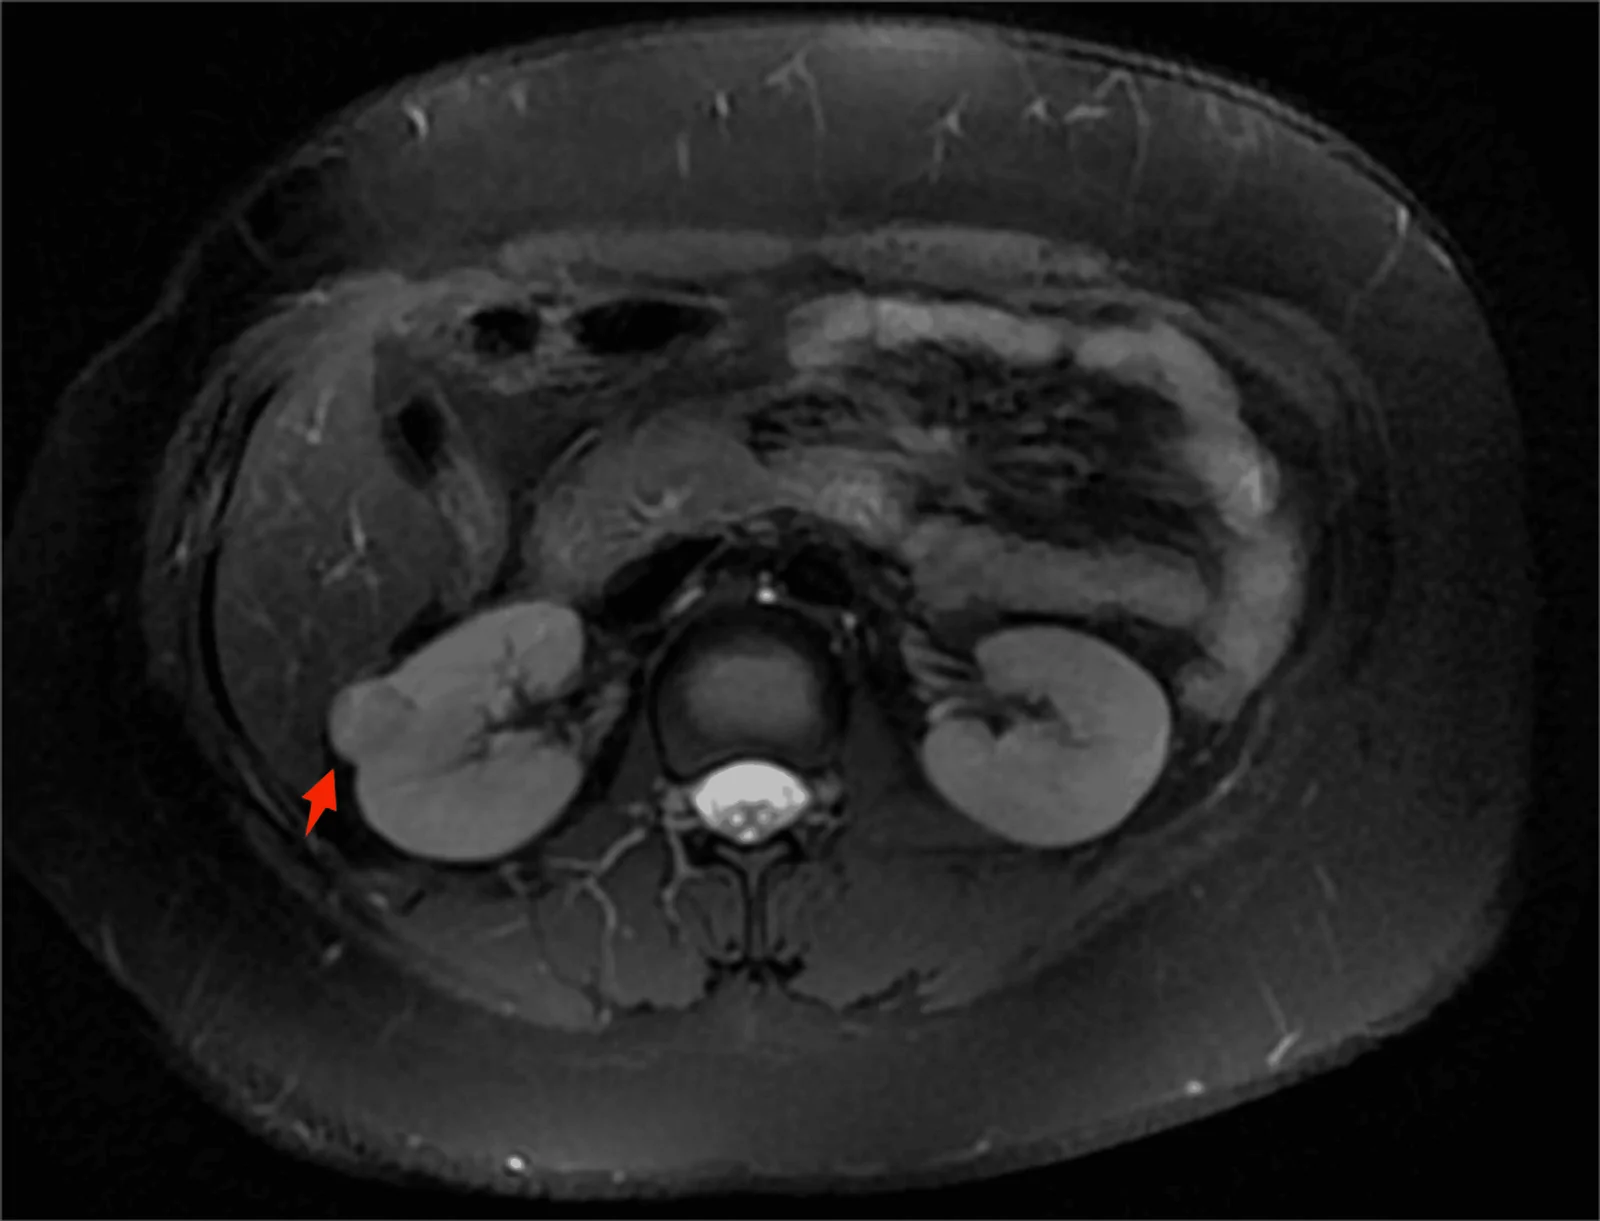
Axial T2WI showing non-uniformity with slightly high signal intensity (arrow) T2WI: T2-weighted imaging
